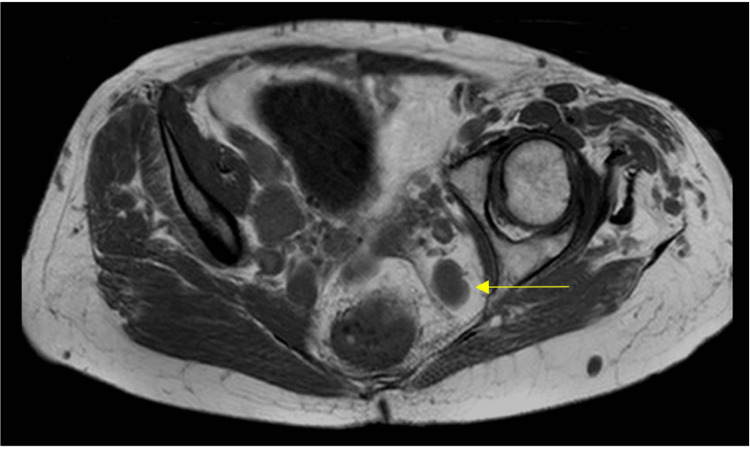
Transverse section of pelvic MRI prior to the first trans-anal repair procedure, showing one of the larger vascular malformations (arrow).
